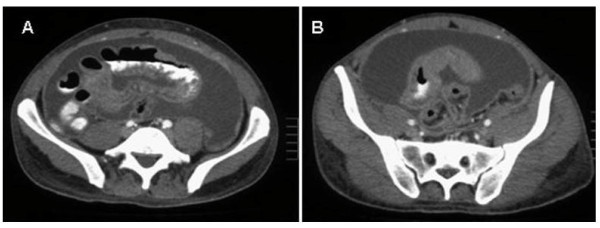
Abdominal CT scan showing: A) infiltration of the mesentery and the peritoneum and B) ascitis and wall tickening of small bowel.
